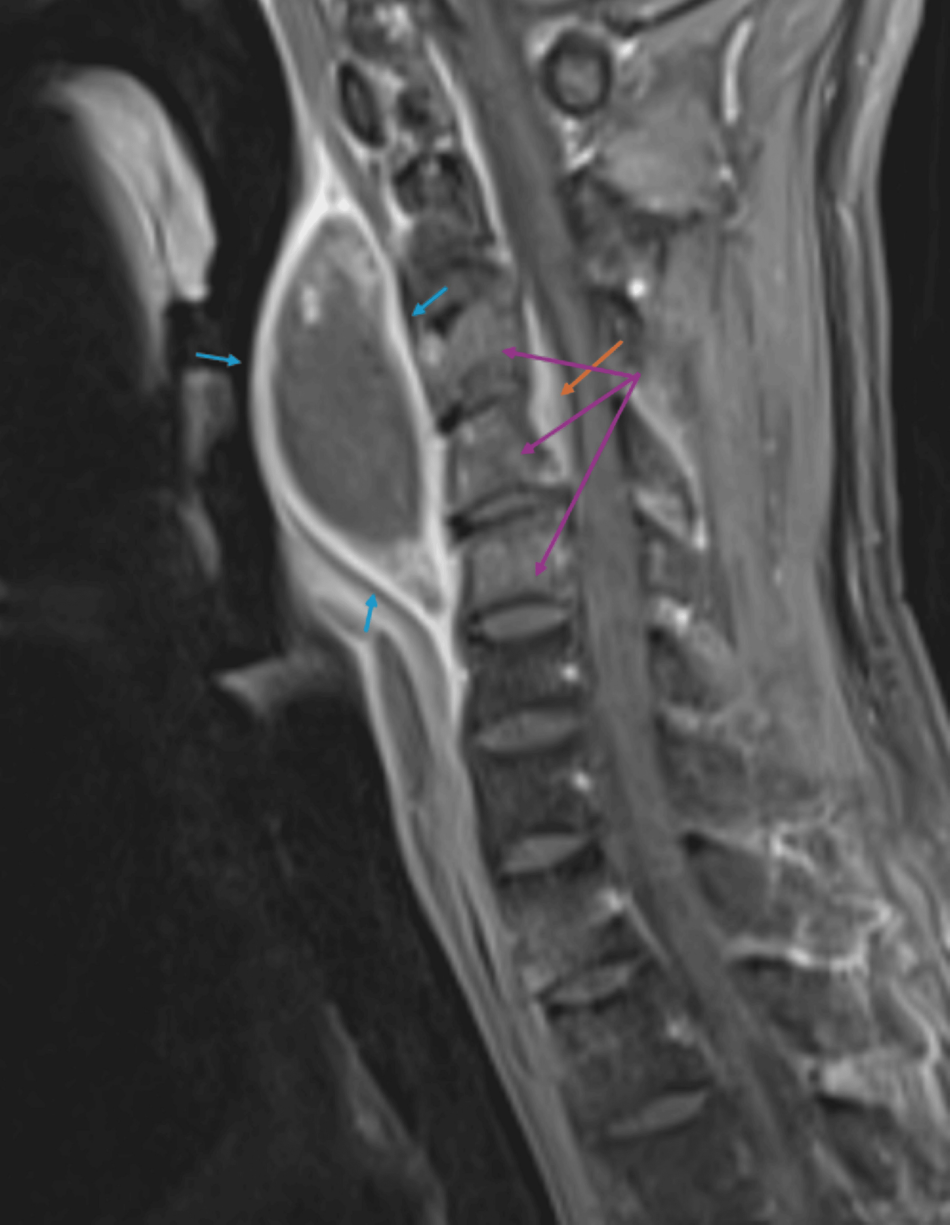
Sagittal contrast-enhanced fat-saturated T1-weighted cervical spine MRI. Findings indicate a homogenous fluid-filled lesion with an enhancing, thick wall, likely representing an abscess between the C1 and C4 vertebrae (blue arrows); enhancing epidural inflammation (orange arrow); and bone marrow enhancement in the C3-C5 vertebral bodies consistent with osteomyelitis (purple arrows) MRI: magentic resonance imaging
